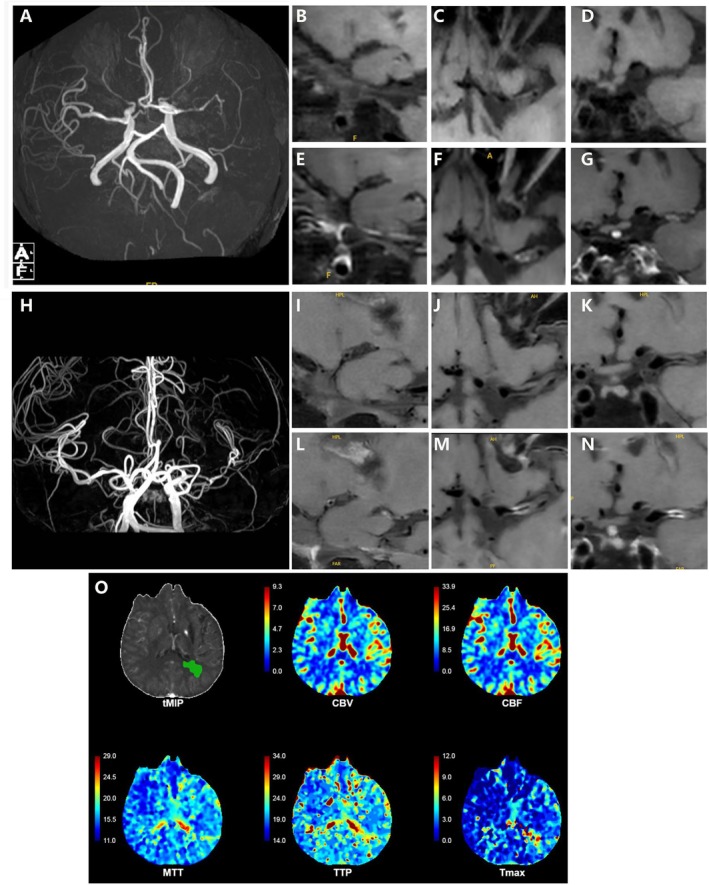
HR‐MRI follow‐ups at 3 T and 5 T, and CTP evaluation 6 months after balloon passage treatment. (A–G) 3 T HR‐MRI images: (A) TOF images; (B–D) sagittal, axial, and coronal non‐contrast images; (E–G), sagittal, axial, and coronal contrast‐enhanced images. (H–N), 5 T HR‐MRI images: (H) 5 T TOF images; (B–D) sagittal, axial, and coronal non‐contrast images; (E–G) sagittal, axial, and coronal contrast‐enhanced images. (O) CTP scan showed significant improvement in left cerebral hemisphere perfusion after 6 months of balloon passage treatment.
